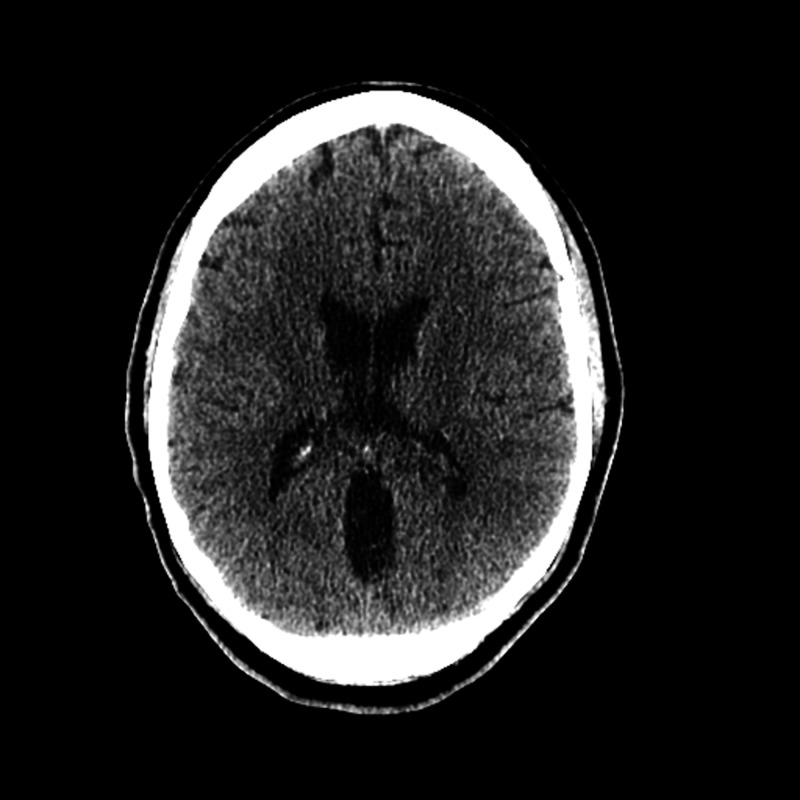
CT of head showing no evidence of intracranial pathology
